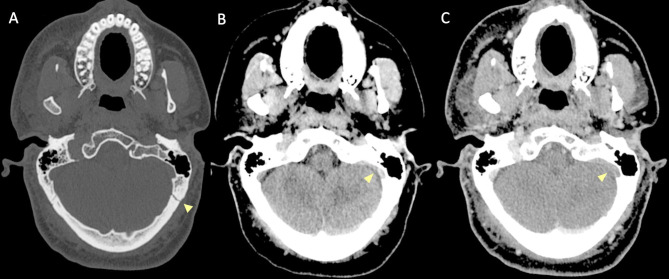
CT scan of a young adult patient involved in a motor vehicle accident. A post-traumatic diastasis of the left occipito-mastoid suture is shown (A, arrowhead) with hyperdensity of the left sigmoid sinus (B, arrowhead). A fracture of the left anterior occipital bone, posterior to the ipsilateral hypoglossal canal is also shown (A). The CTV (C) shows lack of opacification of the left sigmoid sinus, consistent with venous thrombosis (arrowhead)
